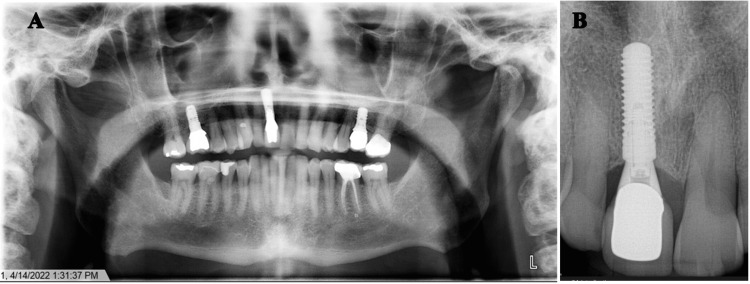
OPG and periapical radiograph A. OPG radiograph showing the implant on tooth #11 is particularly long, but it is not clear if it has any relation with the nasal cavity or the nasopalatine nerve. No destruction or invasion of the adjacent structures. B. Periapical radiograph showing that the implant on tooth #11 is particularly long, 12 mm in length, and has no major odontogenic findings. OPG: orthopantomogram
